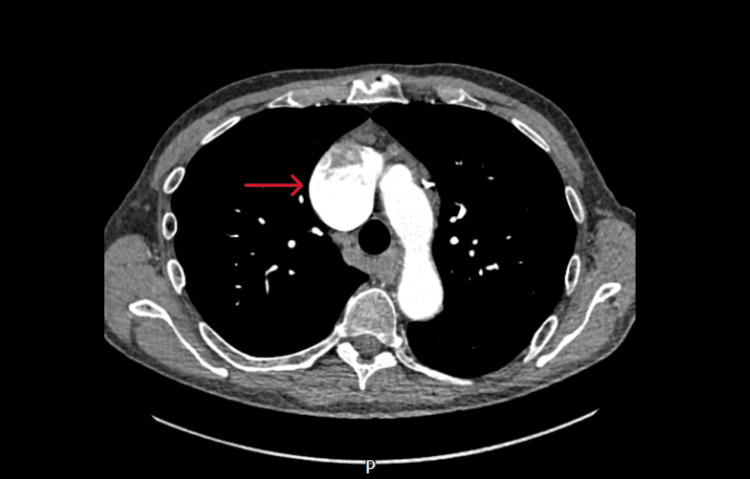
Contrast-enhanced computed tomography image 2 (axial view) The image is showing the large fusiform superior vena cava aneurysm (red arrow).
